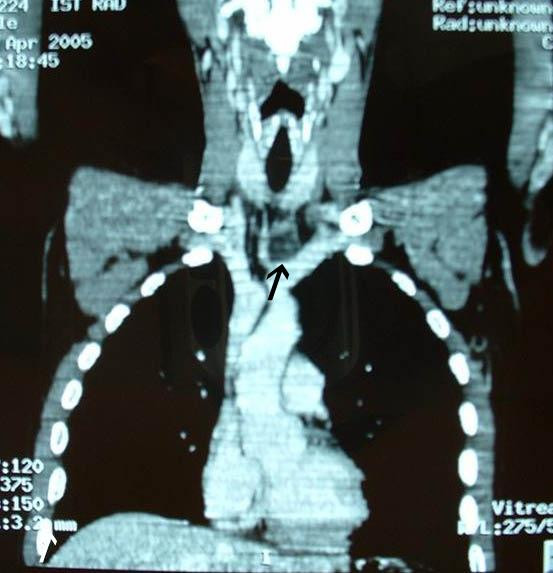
Multislice CT revealed an encapsulated mass of 3 cm in the upper anterior mediastinum behind the sternum-clavicular joints, with marked peripheral enhancement (arrow).
